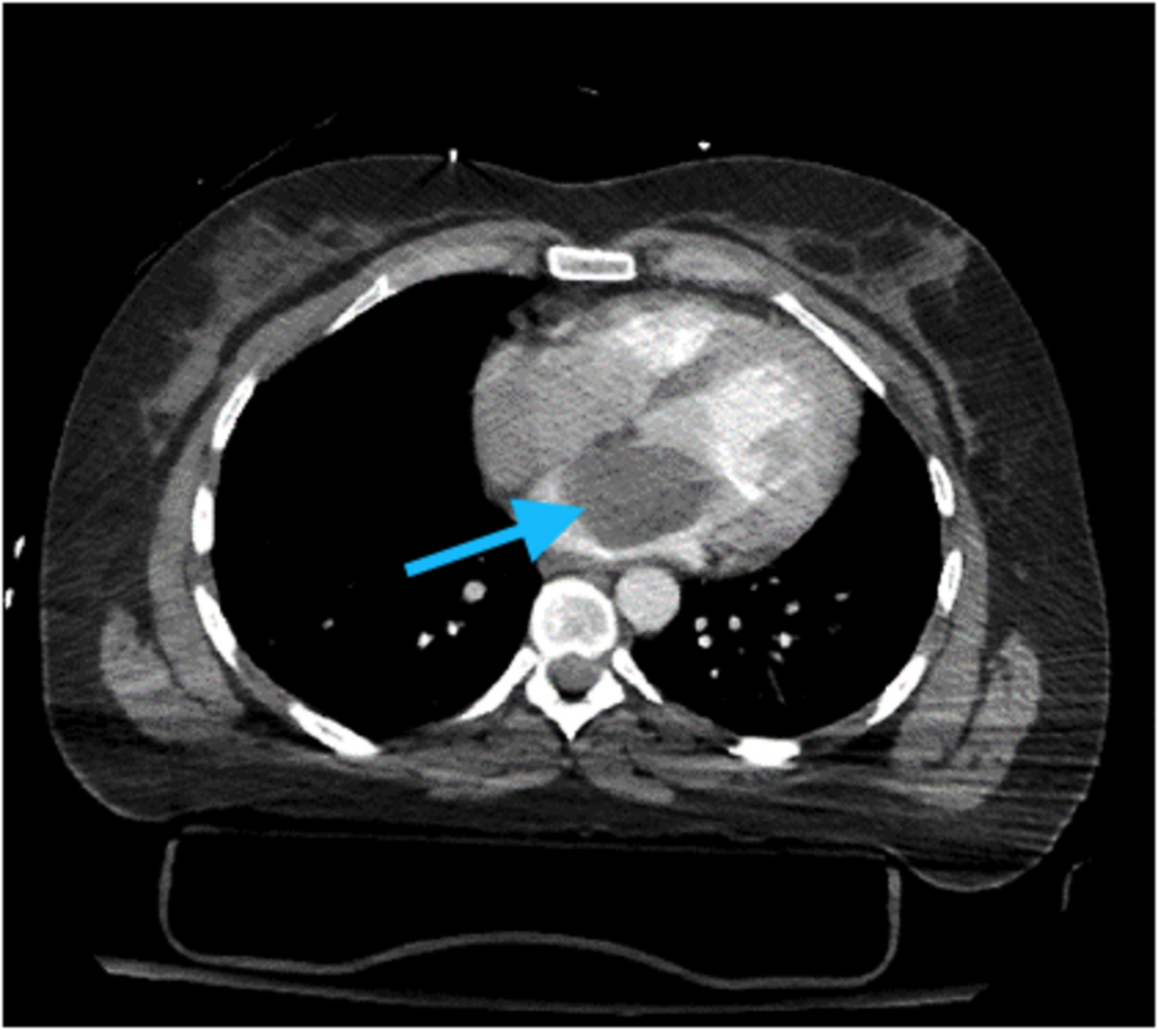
Computed tomography (CT) scan of Chest Blue arrow pointing towards hypodense mass in left atrium
